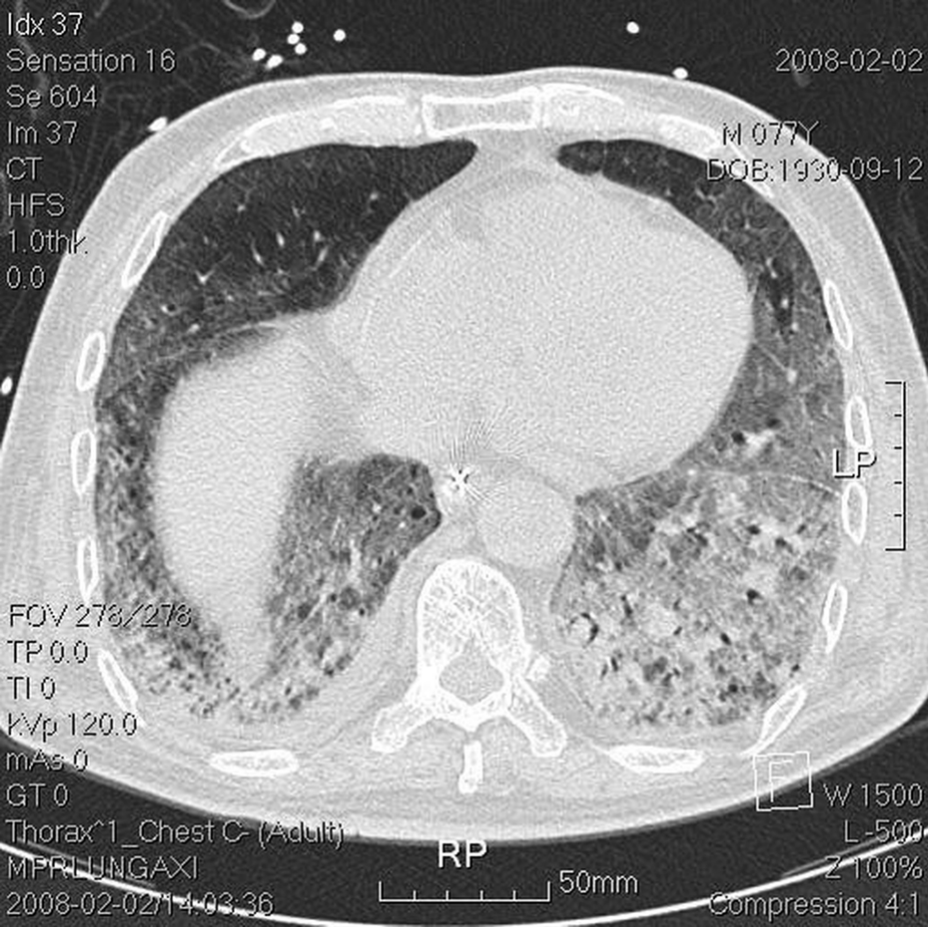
Transverse view of the lower lung field. Chest computed tomography (CT) done post intubation shows ground-glass opacities, blebs confined to the right upper lung apex, and diffuse ground-glass attenuation, blebs in the marginal areas, airspace consolidation, and fibrosis in the bilateral upper and lower lung fields.
